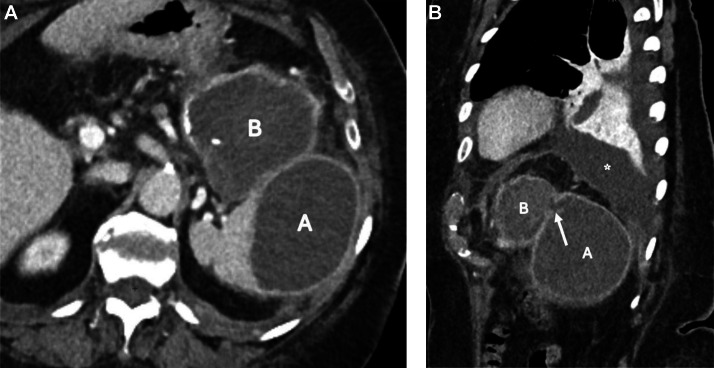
One-month contrast-enhanced CT. Axial image showing splenic subcapsular (A) and perisplenic hematic collection (B) One-month contrast-enhanced CT. Sagittal MPR depicting a fistulous tract (arrow) between the splenic (A) and hematic (B) collections, with associated left pleural effusion (asterisk).
